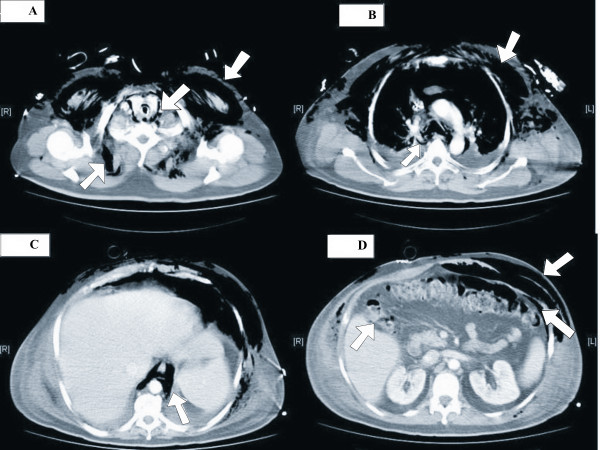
Computed tomography images showing: (A) Subcutaneous emphysema with retrosomatic (lower left arrow) and pectoral musculature gas dissection (upper right arrow), air in the infrahyoid visceral cervical space with separation of thyroid lobe vascular structures (central arrow); (B) Large subcutaneous emphysema in the anterior chest wall (upper right arrow). Gas dissection of the peribronchial cuff in the context of pneumomediastinum (lower left arrow); (C) Presence of air in the retrocrural space. Subcutaneous emphysema, mainly in the anterior chest wall. Pneumoperitoneum with perihepatic distribution (lower right arrow); (D) Subcutaneous emphysema with oblique muscle dissection and anterior pneumoperitoneum (upper right arrows). Small air bubbles below the vesicular bed (upper left arrow).
